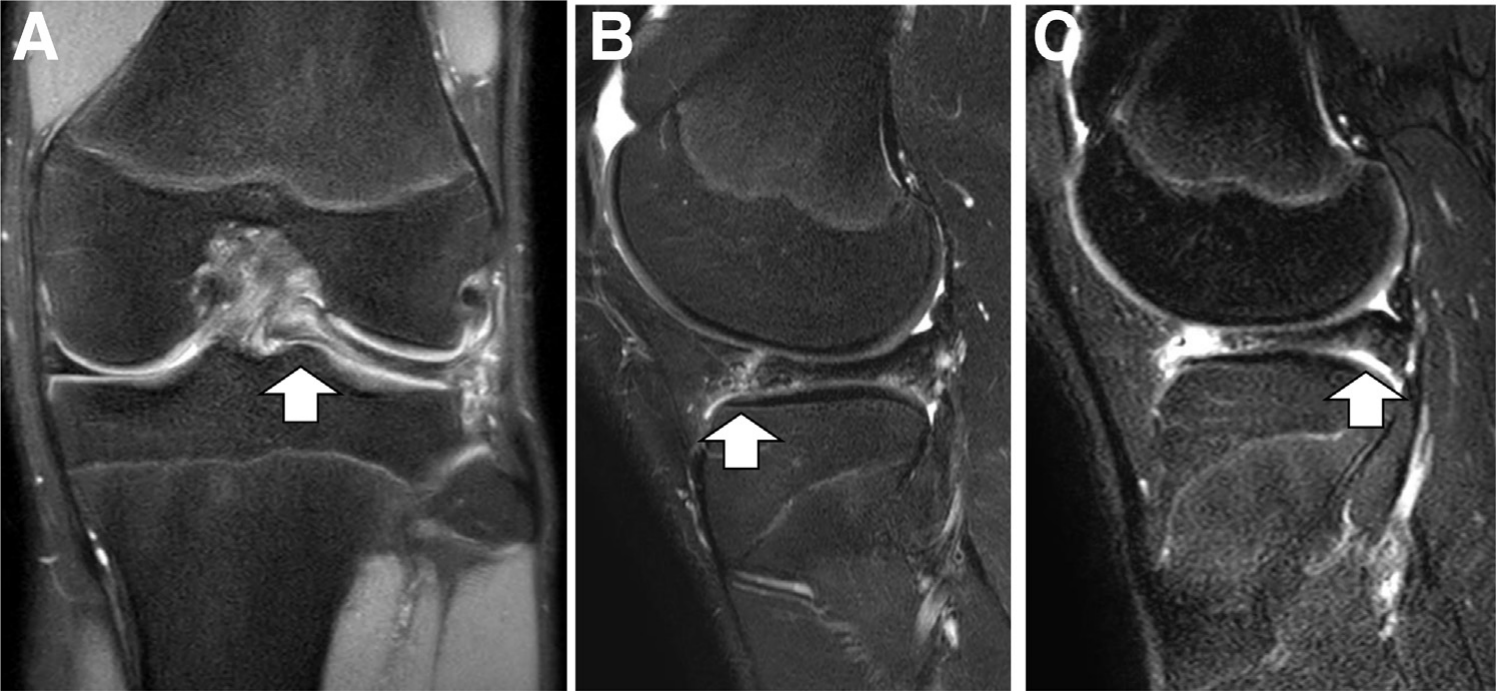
(A) Coronal, (B) sagittal, and (C) sagittal with full-extension knee magnetic resonance images of anterior instability of the discoid lateral meniscus (DLM) in the left knee. The DLM was displaced posterocentrally on the coronal image (A; white arrow), and anterior parameniscal soft-tissue edema was seen on the sagittal image (B; white arrow). The DLM is displaced posteriorly, and the posterior horn appears to be thick on the sagittal images with knee full extension (C; white arrow).
